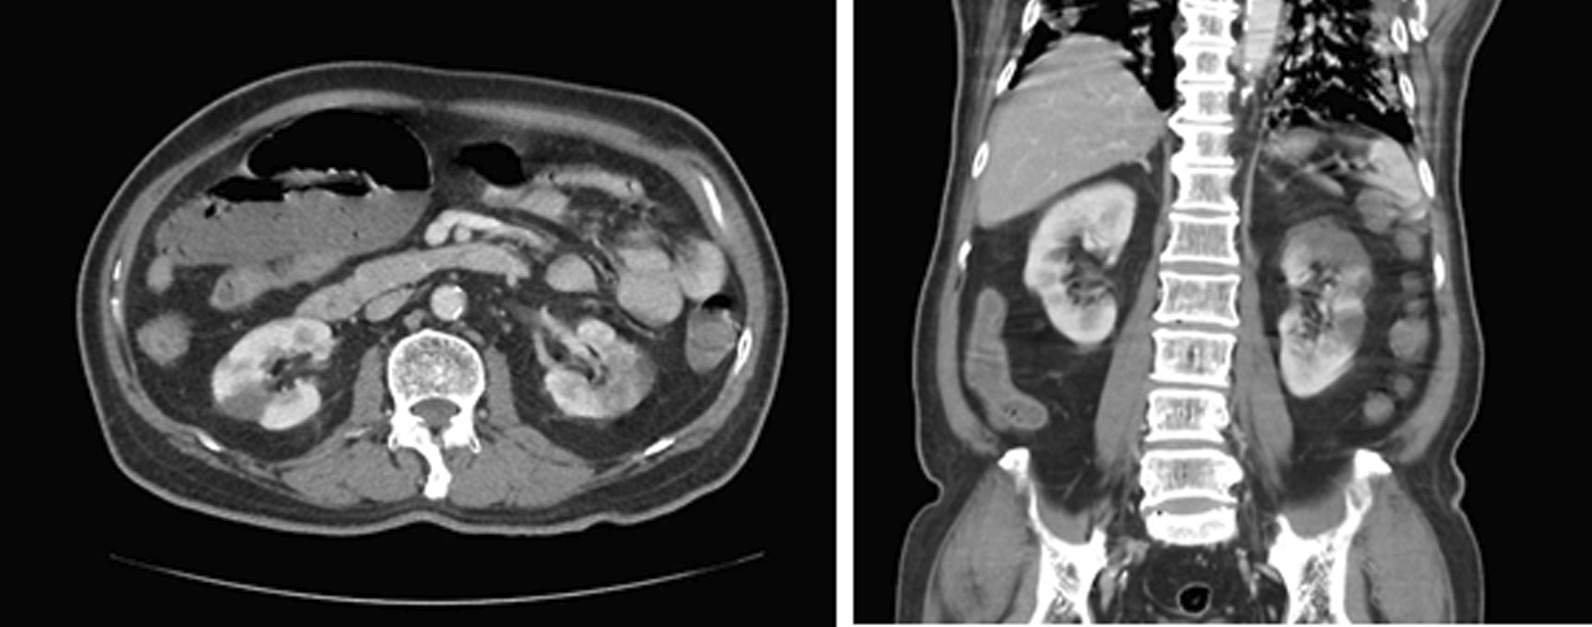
Abdomen CT scan showing bilateral renal infarction
